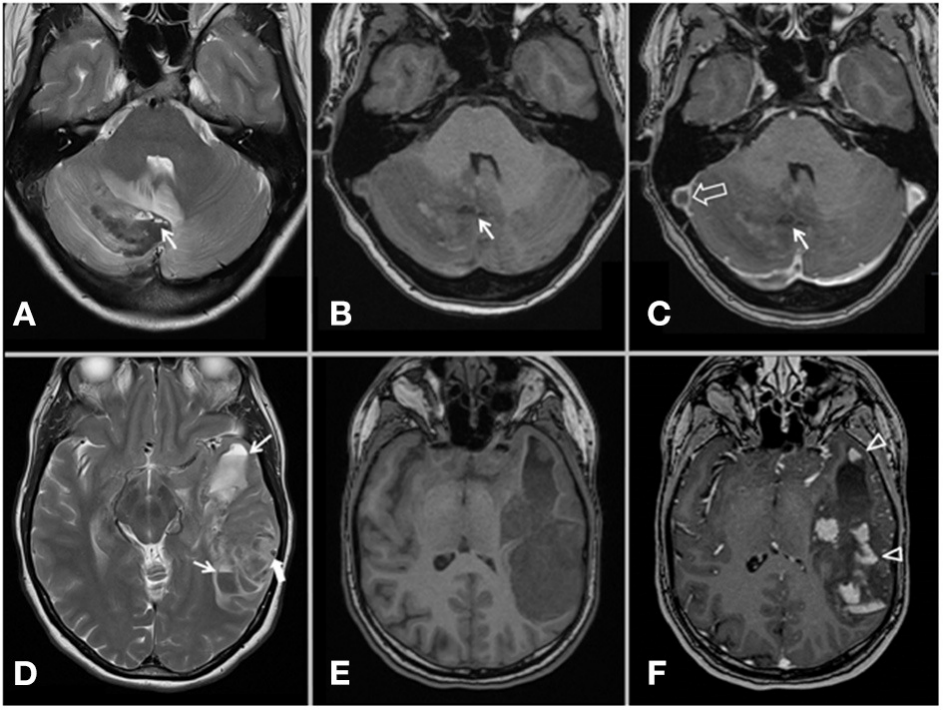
MRI of patient 4 (upper row) and patient 2 (lower row), axial T2-weighted images (A,D), axial T1-weighted images (B,E) and contrast-enhanced axial T1-weighted images (C,F). A complex signal in hematomas of venous infarctions in the right cerebellar hemisphere (patient 4) and left temporal lobe (patient 2) is seen. Multiple fluid-fluid levels (arrows) and sedimentation indicate not fully coagulated blood. Multifocal areas of high signal intensity within the hematoma represents extravasated contrast agent [arrow heads, (F)]. An acute thrombus in the right transverse and sigmoid sinus [open arrow, (C)] cause a well-defined filling defect on the contrast-enhanced T1-MPRAGE. The round lesion with low signal intensity on T2-weighted image [thick arrow, (D)] was tubular on consecutive slices, showed lack of contrast enhancement and is compatible with a cortical vein thrombosis.
